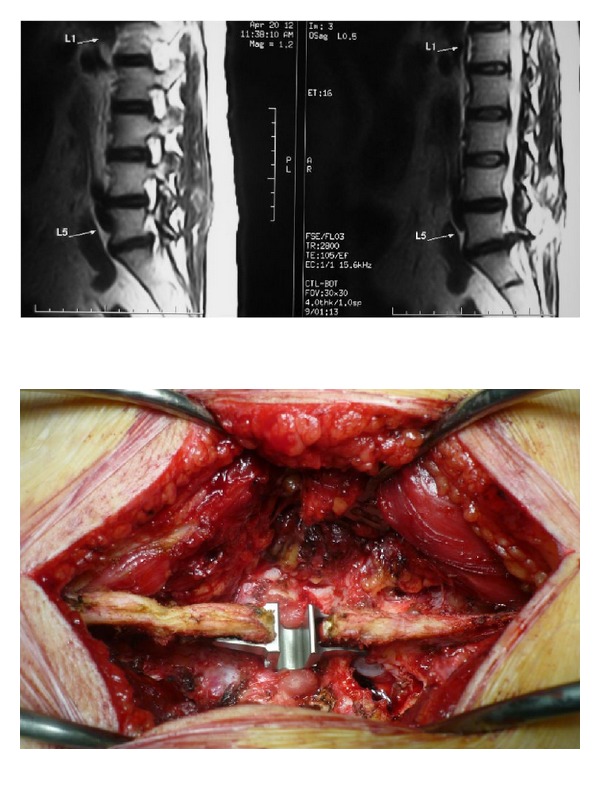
On the left, sagittal T2W image MRI of Lumbosacral spine showing extruded L5-S1 disc with degenerated L4-L5 disc. On the right, preoperative view of the same patient showing left L5-S1 laminotomy for disc excision and L4-L5 interspinous distractor.
